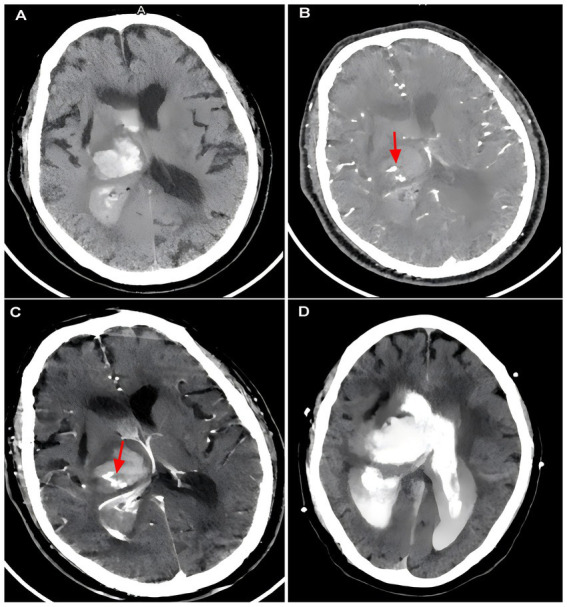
A 79-year-old man with hemorrhage in the right basal ganglia, thalamic-lateral ventricle. The onset time was 4:16 on 10 July, and the time of arrival at the emergency department of our hospital was 6:00. (A,B) first CT and enhanced CT at 6:12, hematoma volume was 9.45 mL, spot sign positive (red arrow); (C) sequential CT scan at 6:16 with positive brush sign and continuous morphology (red arrow); and (D) re-examined CT at 9:53, and the hematoma volume was 20.20 mL, which showed obvious hematoma expansion.
